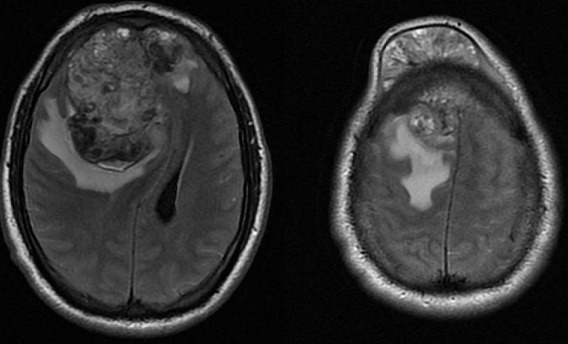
T2 MRI sequence that shows a large metastatic intra and extracranial right frontal lesion
